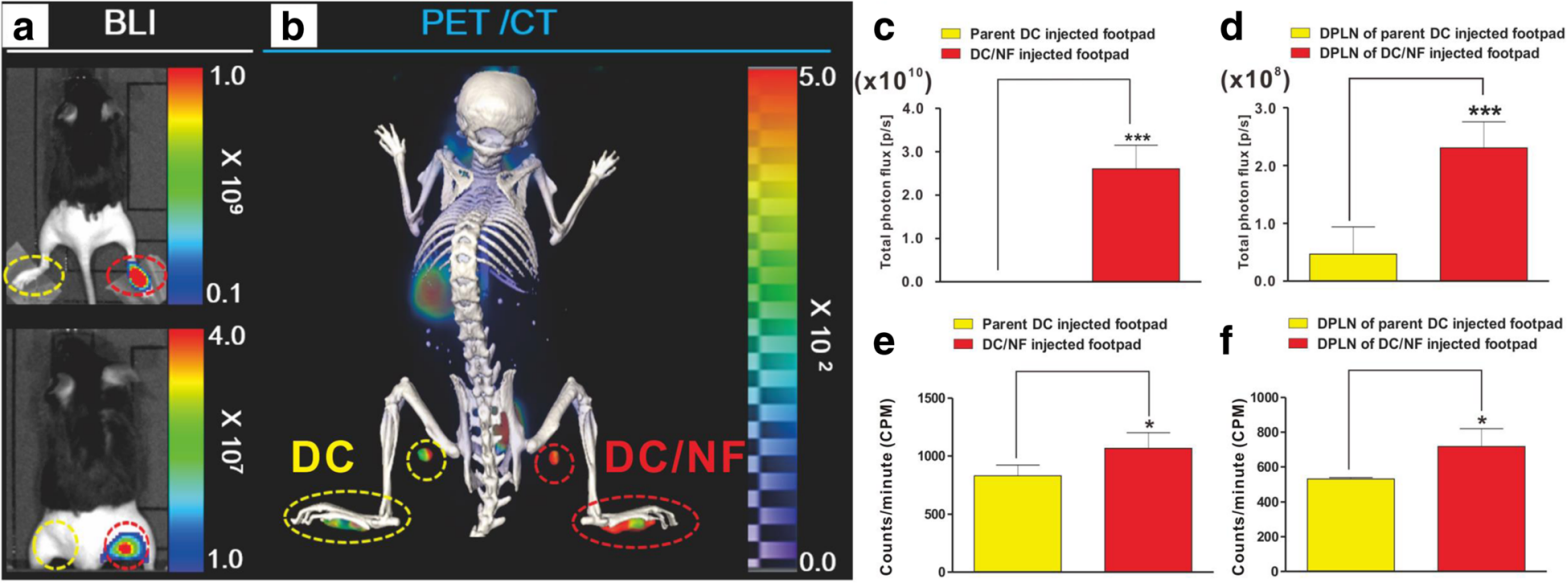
In vivo combined BLI and ^18^F-TFB PET/CT imaging of DC migration after their subcutaneous injection into footpads. a In vivo BLI (*upper panel*: without masking the footpads; *lower panel*: after masking the footpads) and b
